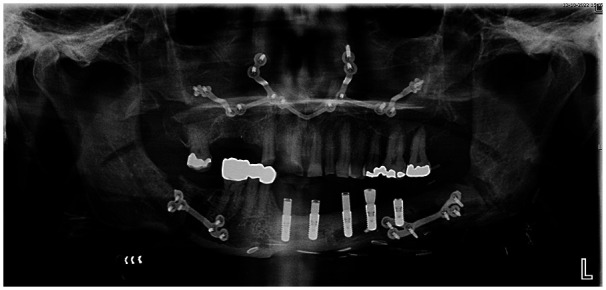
Panoramic radiograph two weeks after maxillomandibular advancement.
